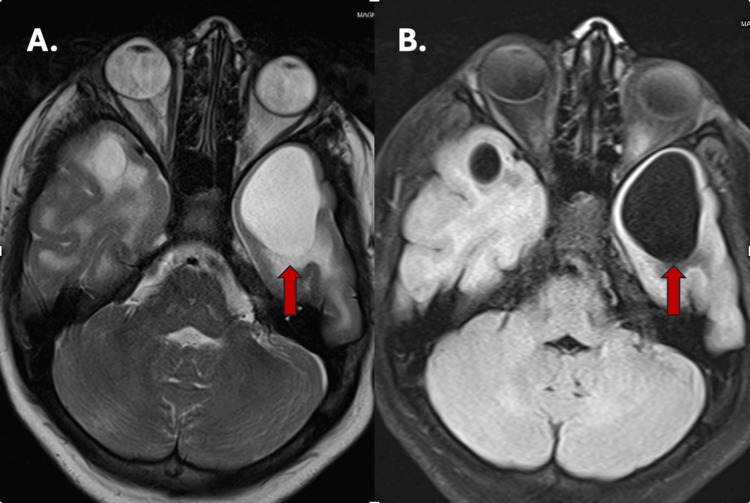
(A) Axial T2W 3-T and (B) axial FLAIR 3-T MRI images demonstrating classical subcortical temporal cysts (red arrows) showing complete suppression on FLAIR sequences. T2W, T2 weighted; FLAIR, fluid-attenuated inversion recovery
